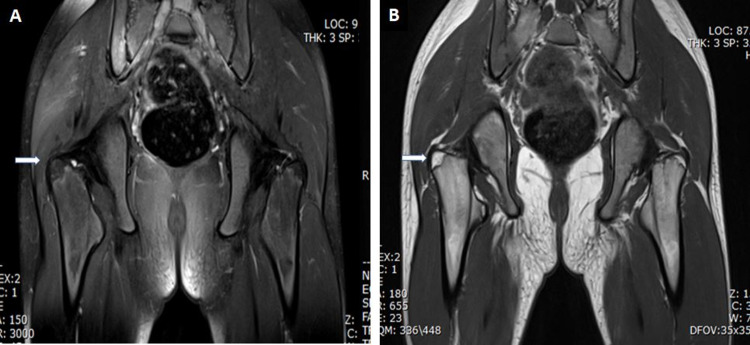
MRI pelvis with contrast A. Selected coronal proton density (PD) fat-sat image showing low signal intensity lesion involving the greater trochanter of the right femur (white arrow). B. Selected coronal T1-weighted image showing high signal intensity, well-defined lesion in the same region (white arrow) MRI: magnetic resonance imaging
